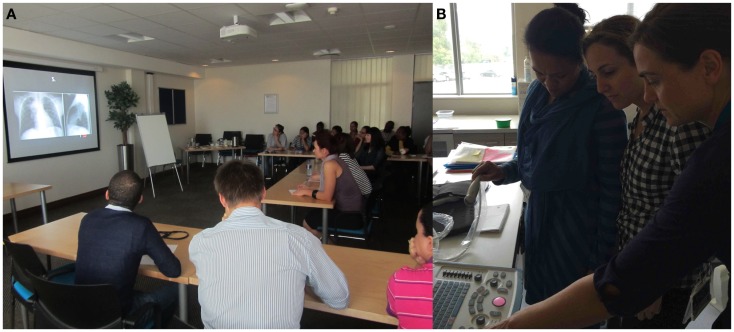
Training of non-radiologists to interpret radiographs is part and parcel of telereading support and capacity development. (A) A large group of family physicians participating in a pre- and post-training, pediatric X-ray reporting, self-assessment session, hosted by the visiting outreach radiologist from the South African Society of Pediatric Imaging acting (under the auspices of the World Federation of Pediatric Imaging). This program was run twice in parallel with the pilot telereading program, intended to increase capacity for X-ray interpretation on the ground. (B) Point of care mediastinal sonography for the diagnosis of tuberculosis being taught to a non-radiologist by a representative of Imaging the World in co-operation with the World Federation of Pediatric Imaging.
